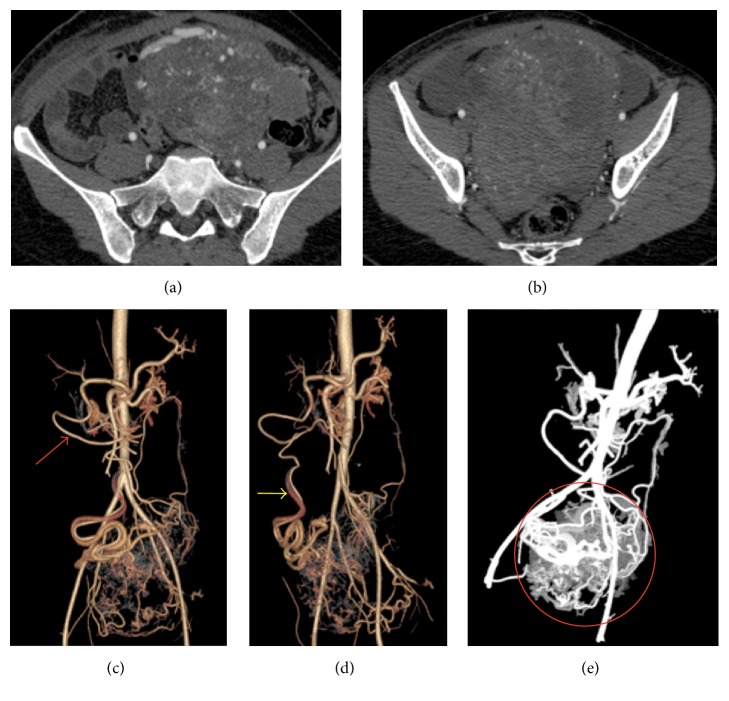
CT Angiography. Axial scan through the lower abdomen revealed a large heterogenous mass with multiple intralesional vascular channels ((a) and (b)); volume rendering reconstructed images ((c) and (d)) show a hypertrophied vascular channel (omental branch) arising from the right gastroepiploic artery (red arrow on (c)) which is encircled with a draining tributary which is drained into the superior mesenteric vein (yellow arrow on (d)); an angiographic reconstructed image (e) shows a highly vascular mass with multiple intralesional vascular channels (red circle).
